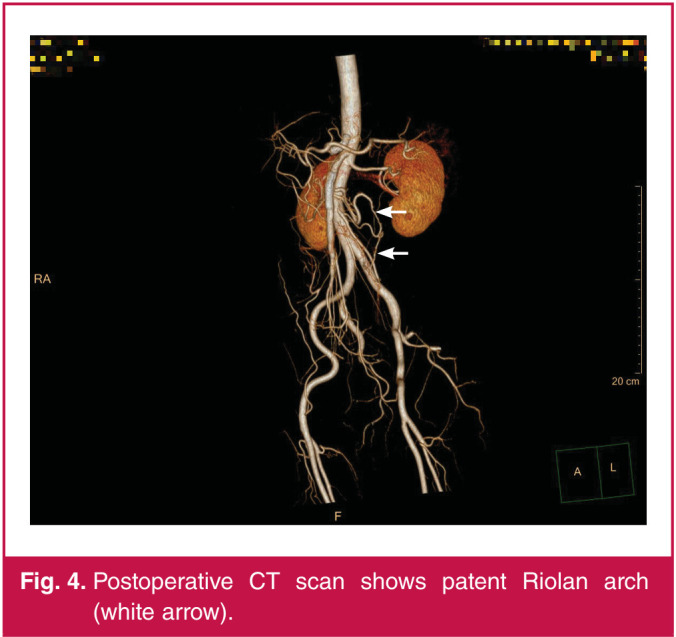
Postoperative CT scan shows patent Riolan arch (white arrow).
